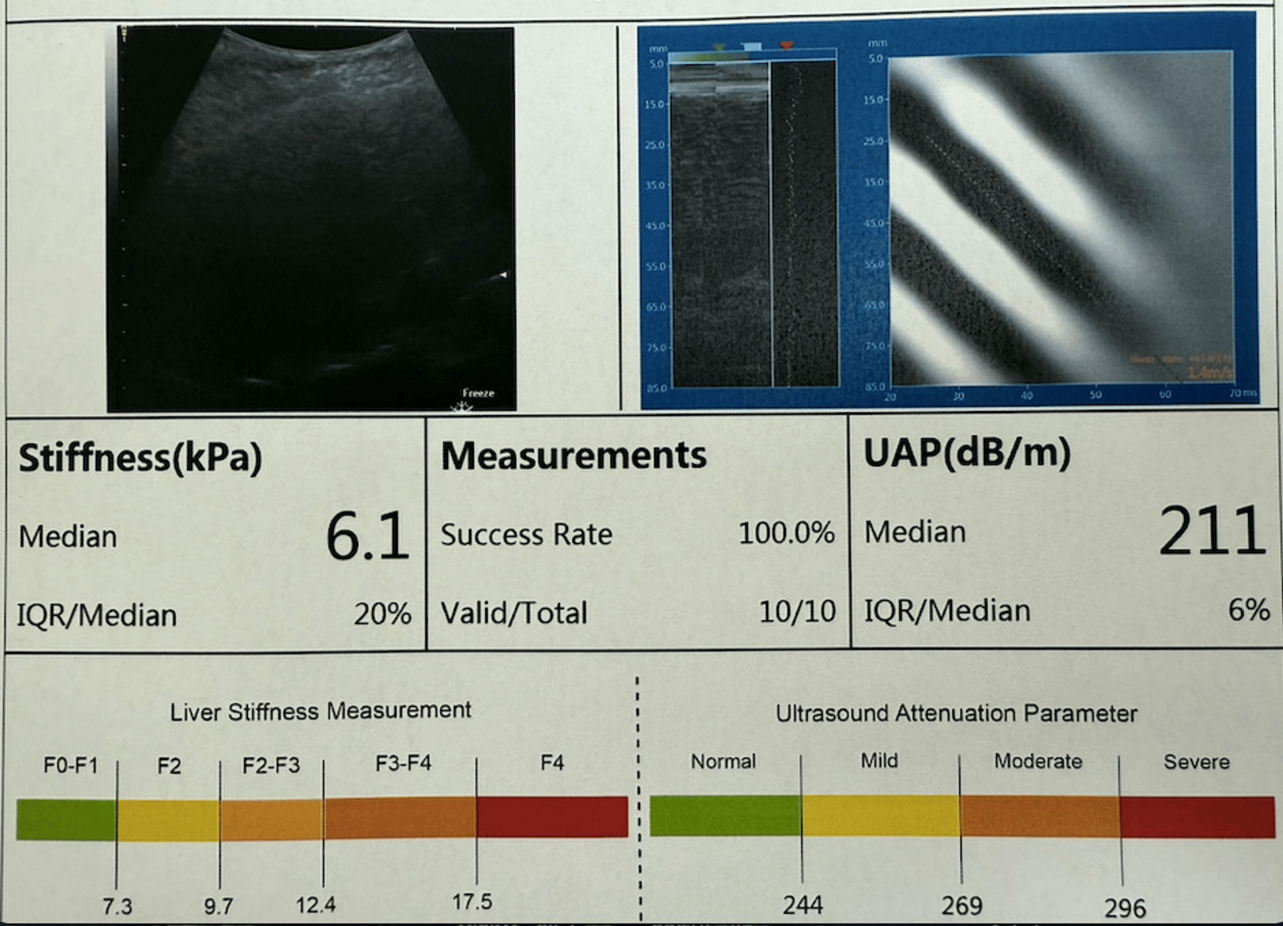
Liver elastography at one year Liver elastography showed F0 fibrosis with a stiffness of 6.1 kPa and a normal ultrasound attenuation parameter of 211 dB/m, indicating the absence of hepatic steatosis.
